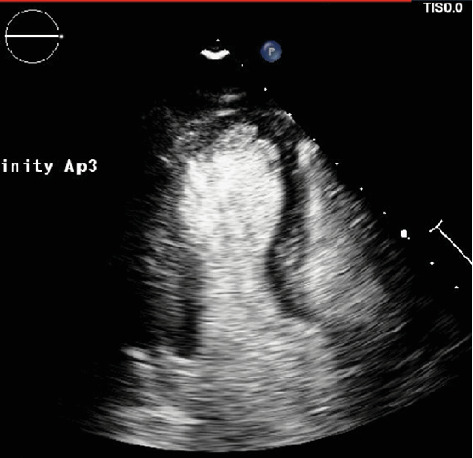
Apical 2 chamber echocardiogram with definity contrast.
